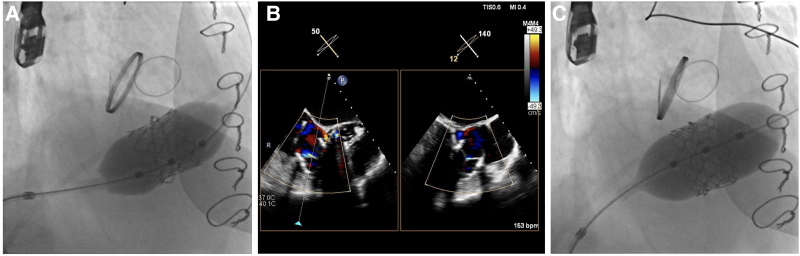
(*A*) Once the valve was correctly positioned, it was expanded to fit inside the tricuspid annuloplasty ring. (*B*) Control transoesophageal echocardiography showing a moderate paravalvular leak. (*C*) Post-dilation of the valve to reduce paravalvular leakage.
